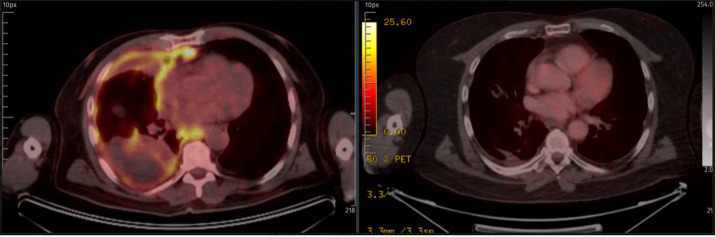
Baseline and post-treatment PET-CT showing complete metabolic response.
